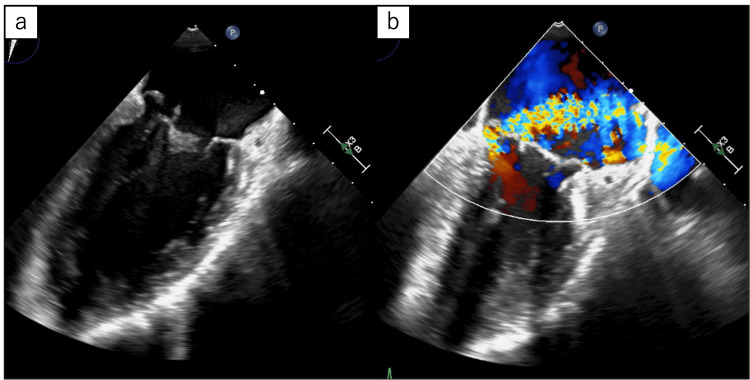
Transesophageal echocardiogram showing (a) several irregularly shaped hypoechoic masses approximately 4 mm in size on the mitral valve leaflets, and (b) moderate mitral regurgitation.
